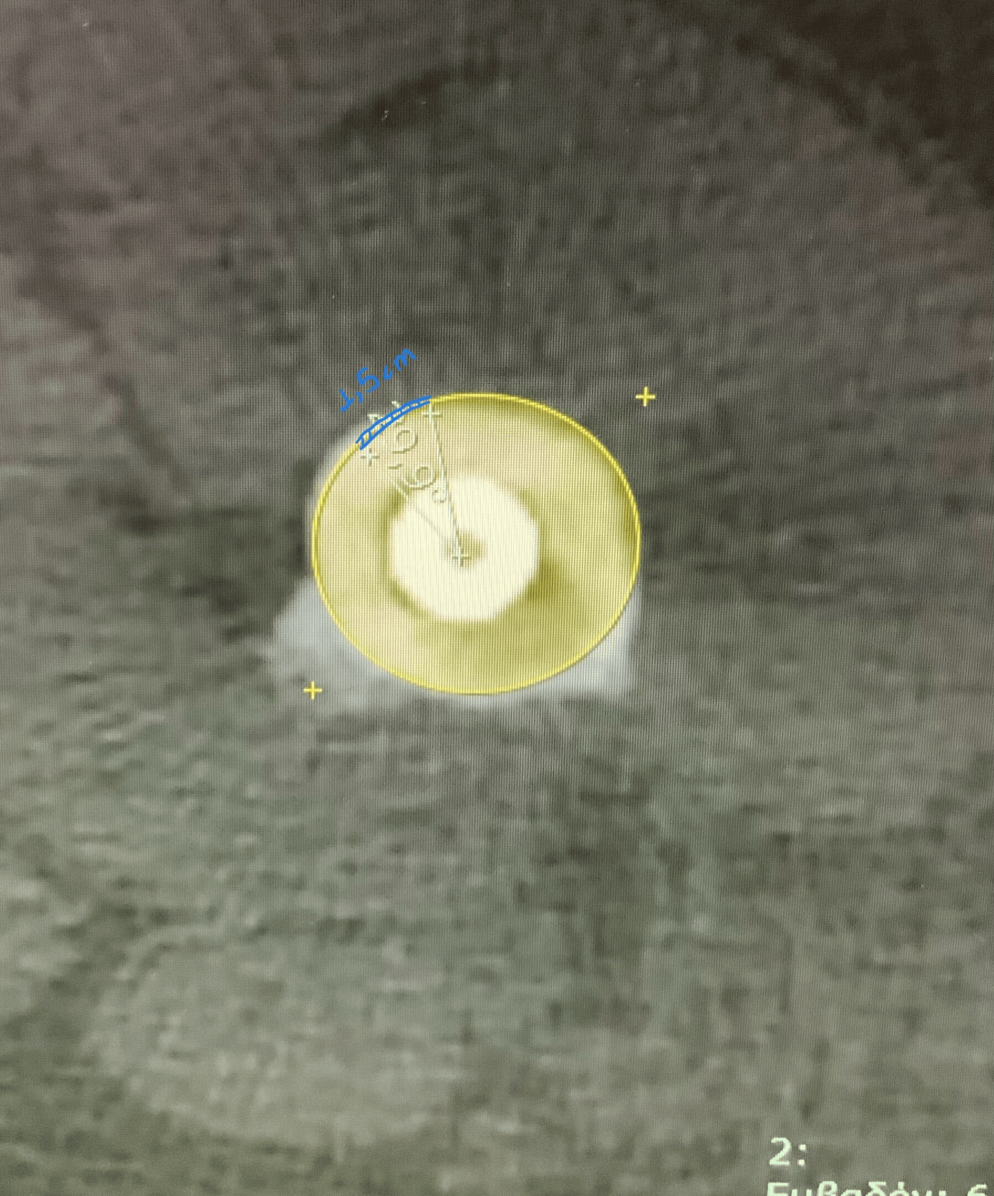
Preoperative planning Axial image from the same CT scan showing the 30° angle measured at the osteotomy level and the corresponding translational distance.
